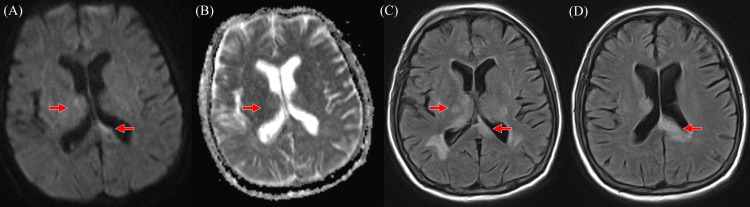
(A) Axial DWI shows subtle hyperintensity corresponding to posterior thalami and posterior periventricular white matter with inconsistent (B) ADC hypointensity and corresponding (C) axial FLAIR image at thalami and (D) splenium Images subject to motion-degradation. *Abbreviations: *ADC = Apparent Diffusion Coefficient, DWI = Diffusion-Weighted Imaging, FLAIR = Fluid-Attenuated Inversion Recovery
